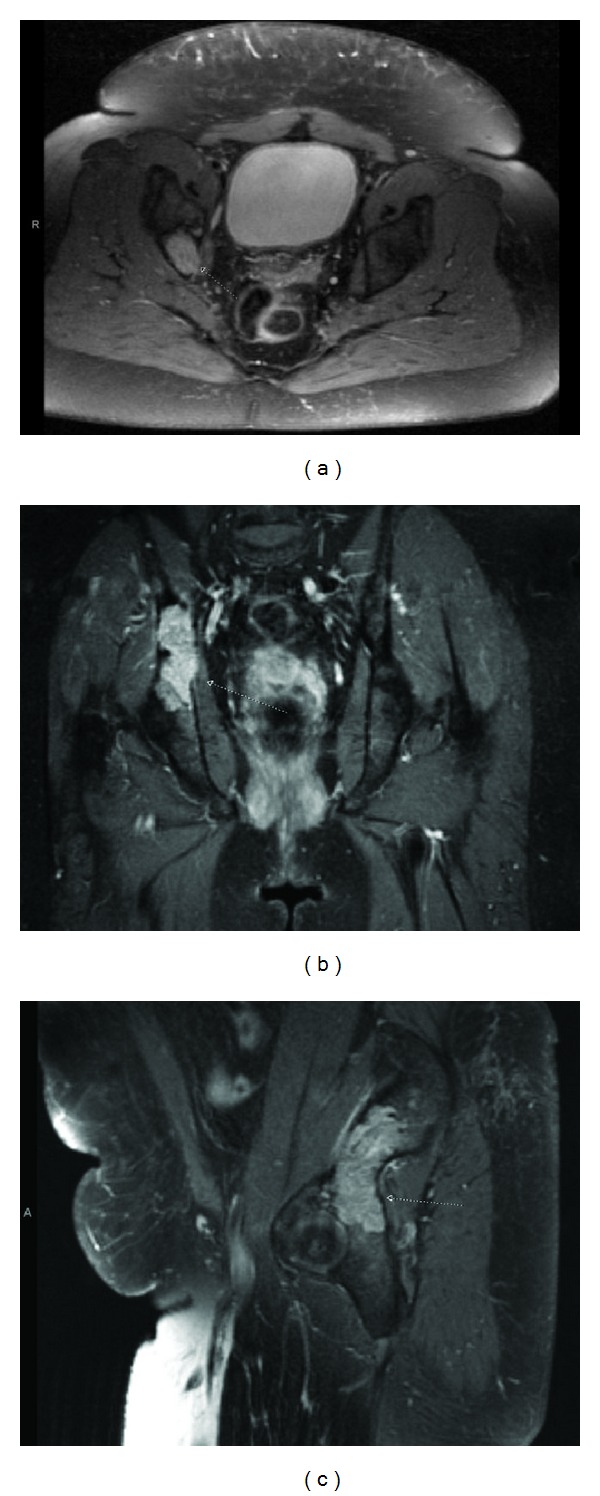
Preradiotherapy axial (a), coronal (b), and sagittal (c) postcontrast T1-weighted magnetic resonance imaging scans showing a right iliac heterogeneously enhancing mass.
